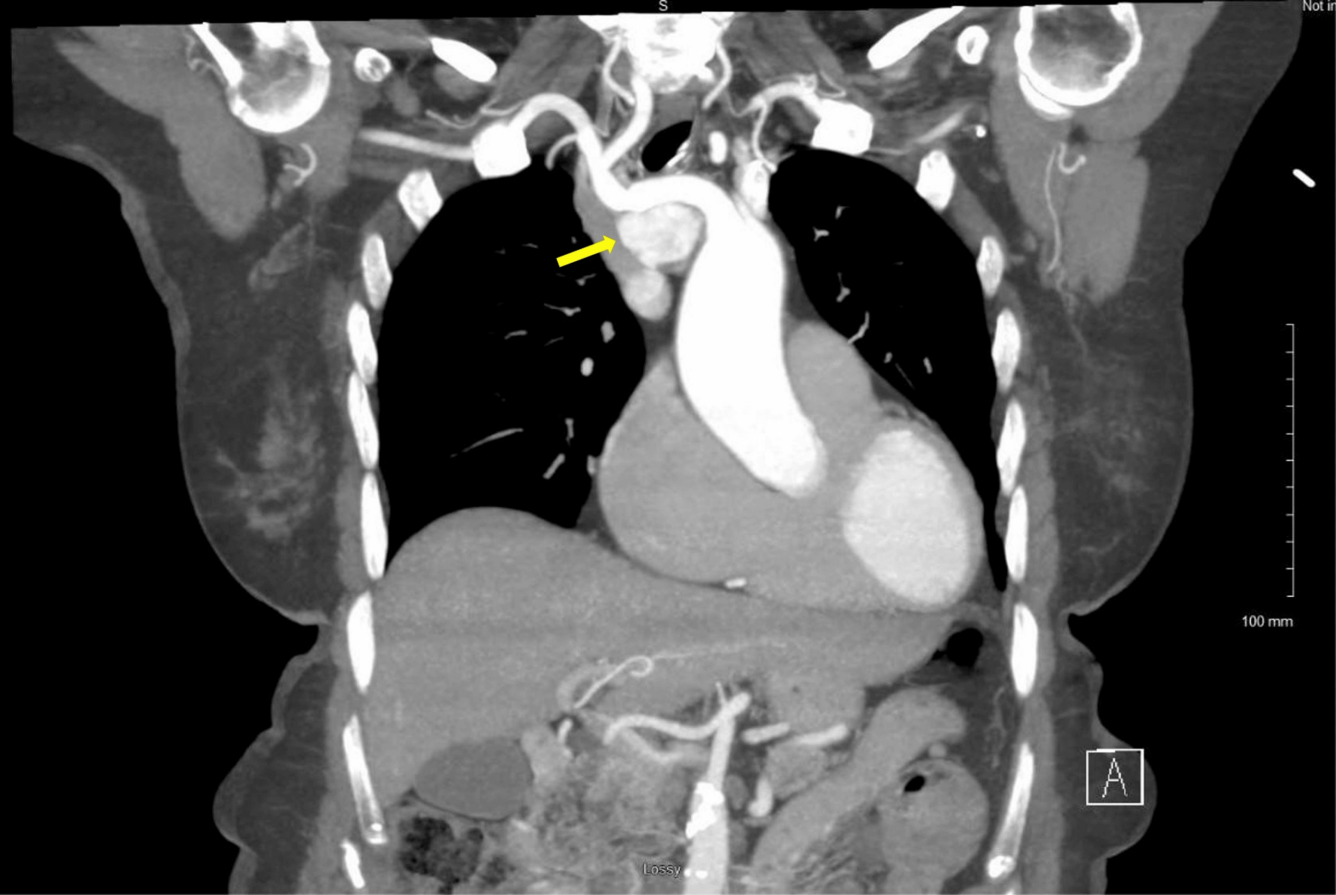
Coronal view of a preoperative computed tomography of the chest with IV contrast demonstrating a right paratracheal enhancing mass (yellow arrow) and its intimate proximity to the great vessels of the mediastinum.
